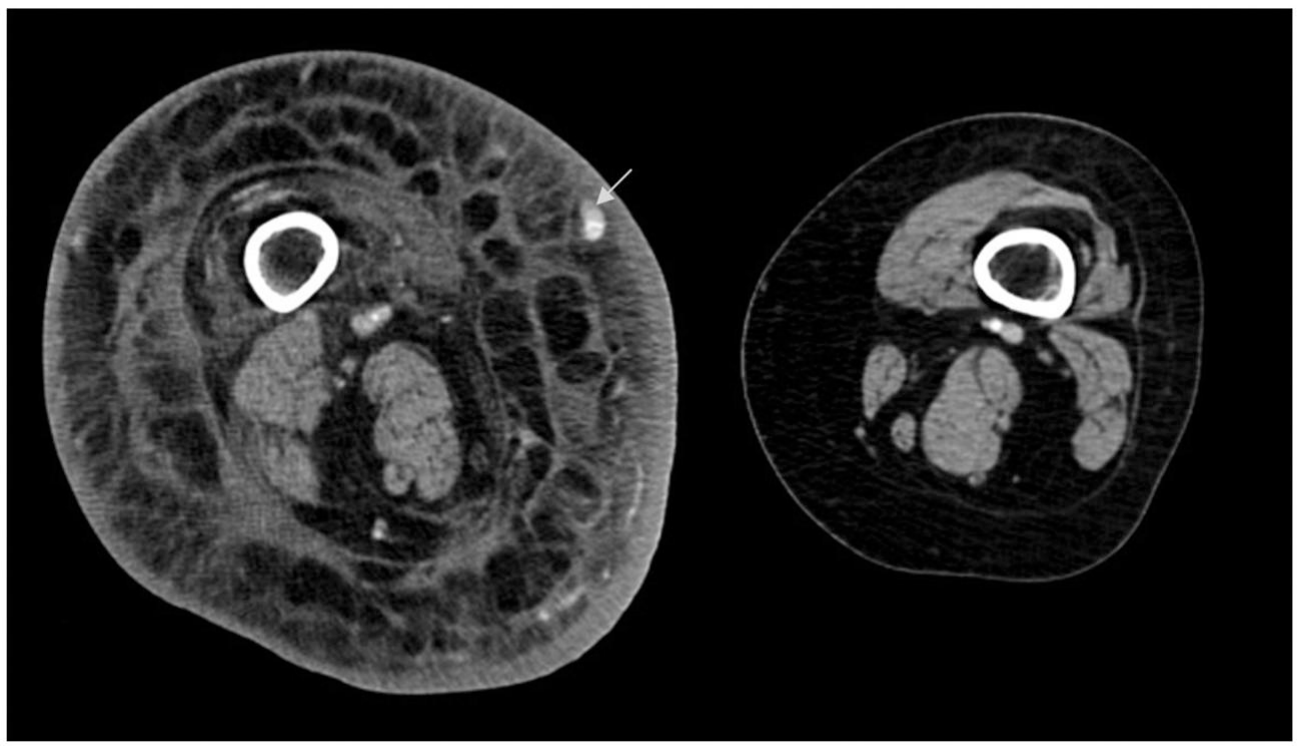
Contrast-enhanced CT scan of bilateral lower limbs of a patient with a right thigh synovial sarcoma, who developed swelling in the right lower limb, a year after surgery and radiotherapy. Note the diffuse skin thickening and cobblestone appearance of the subfascial and epifascial plane, due to the fluid and the fibrosis surrounding the fatty tissue. Prominent venous collaterals are also seen in the subcutaneous plane (arrow). The contralateral limb appears normal.
